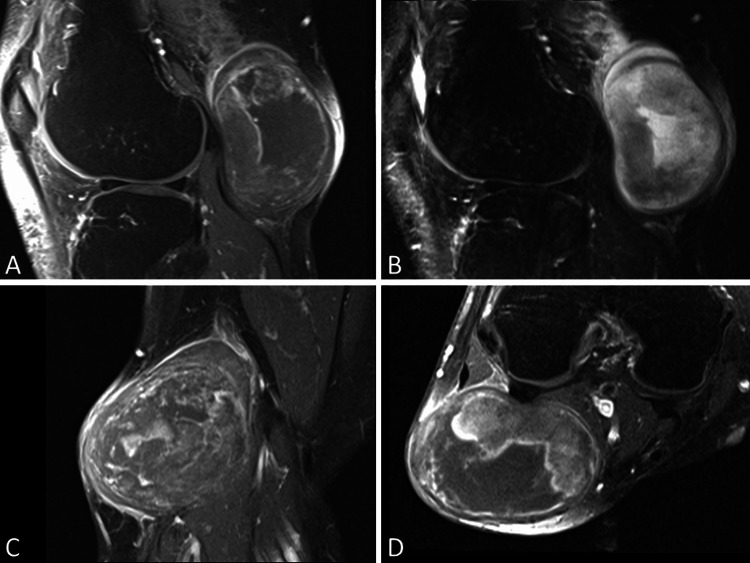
MRI characterization of the tumor within the peroneal nerve MRI revealed a 7 cm well-defined mass lesion within the peroneal nerve. The lesion exhibited mildly low signal intensity in the T1 sequence with contrast enhancement (A) and high signal intensity with peripheral edema in the T2 sequence (B). The coronal (C) and axial (D) view showed core region heterogeneity of the mass.
